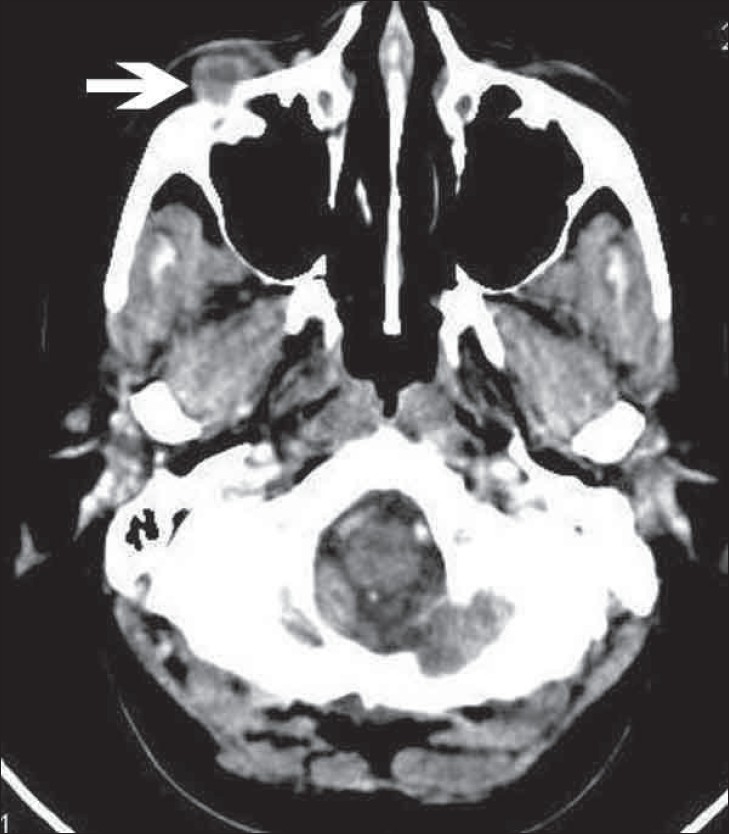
Post-contrast axial CT image showing heterogeneous enhancement of the lesion (arrow)
